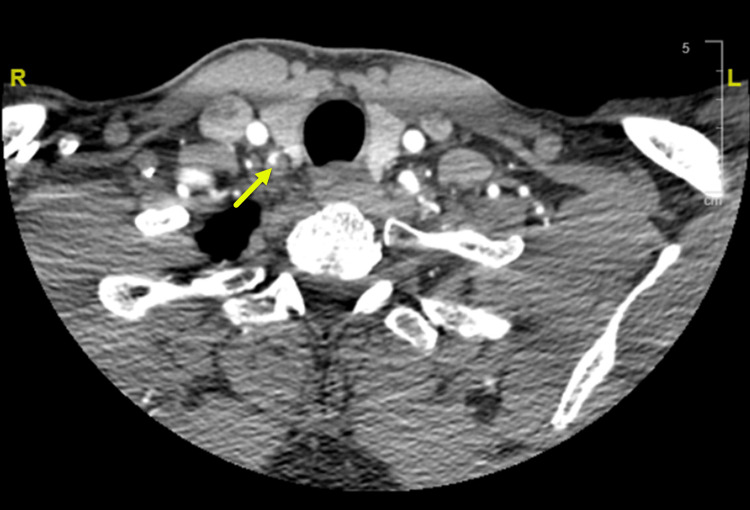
Right vertebral artery stenosis CT angiogram (CTA) of head and neck with contrast showing the circumferential noncalcified plaque of the V1 segment proximal right vertebral artery causing severe, greater than 90% stenosis. The left vertebral artery is patent. The vertebral arteries are symmetric in size, both contribute to the basilar artery.
